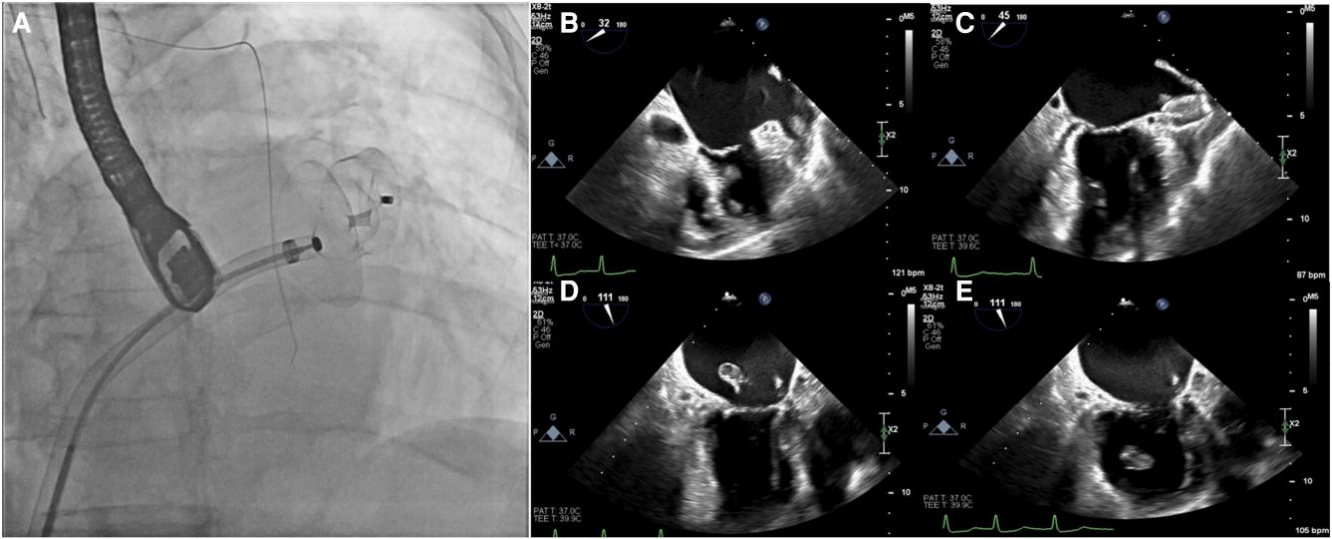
Intraprocedural transoesophageal echocardiography. (*A*) Left appendage occlusion device deployment. (*B*) Thrombus formation in the left atrial appendage before occlude deployment. (*C*) Thrombus attached to occlude device. (*D*) Mobile thrombus in left atria. (*E*) Thrombus advancing to left ventricle with consequent systemic embolism.
